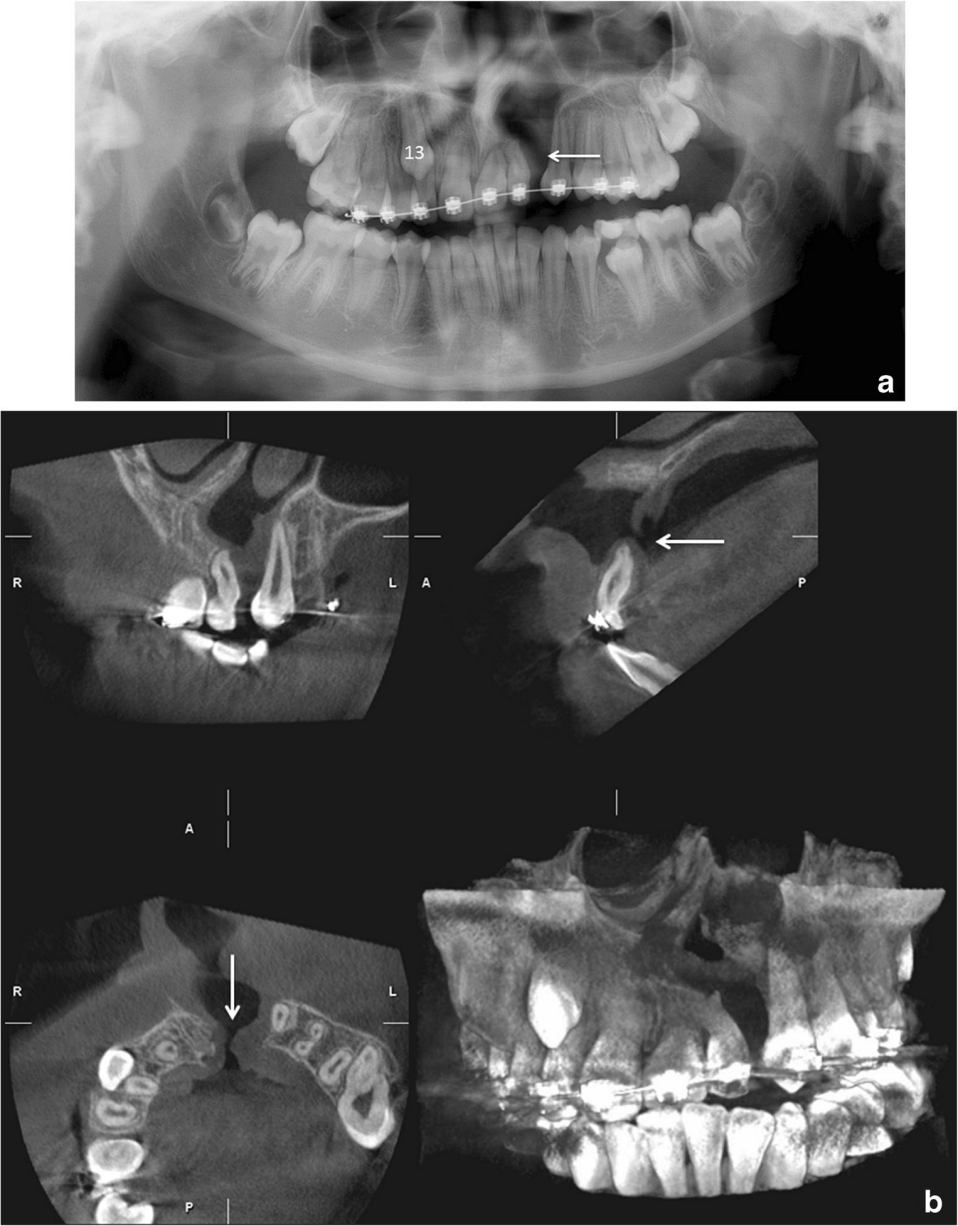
a A patient with a unilateral cleft lip and palate has an alveolar defect on the left side (*arrow*). A PTG image taken before secondary alveolar bone grafting (*SABG*). Note also crowding in region d. 13, which can also be easily evaluated in the CBCT examination. b CBCT for the treatment planning before SABG allowing evaluation of the bone defect with oronasal fistula (*arrows*)
